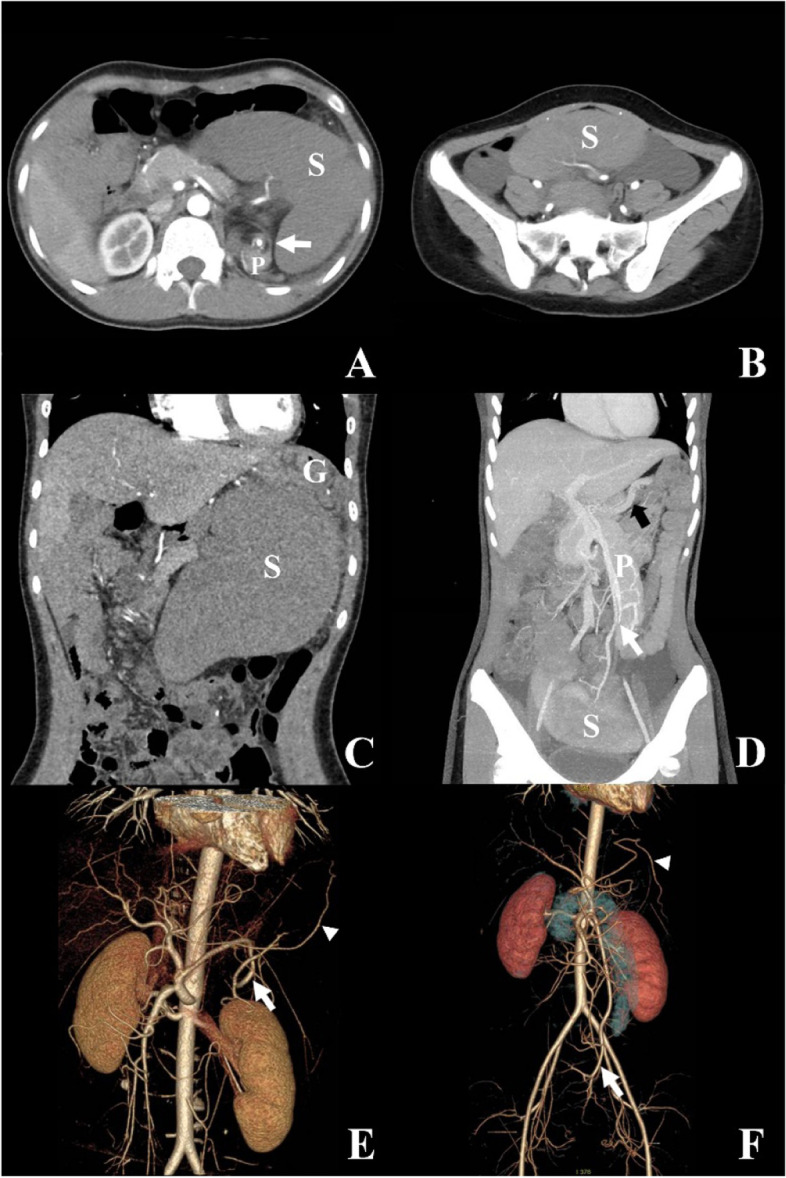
Comparison of two preoperative contrast CT results with 6 days interval in case 5, showing the spleen moving from left hypochondrium to the pelvis. A&B: The splenic enhancement was reduced in A and was partially enhanced in B. (S = spleen, *P* = pancreas, white arrow shows “Whirl sign” with enhanced splenic artery in the center and pancreatic tail involved.). C&D: Coronary views show normal location of gastric fundus in C (G = gastric fundus), splenic vein interruption in D (white arrow, indicating thrombosis) and engorged left gastric vein in D (black arrow). E&F: Artery reconstruction views show a splenic artery (white arrow) circling (indicating torsion) in E and running downward in F, and route change of gastro-epiploic artery (white triangle, indicating gastric volvulus)
